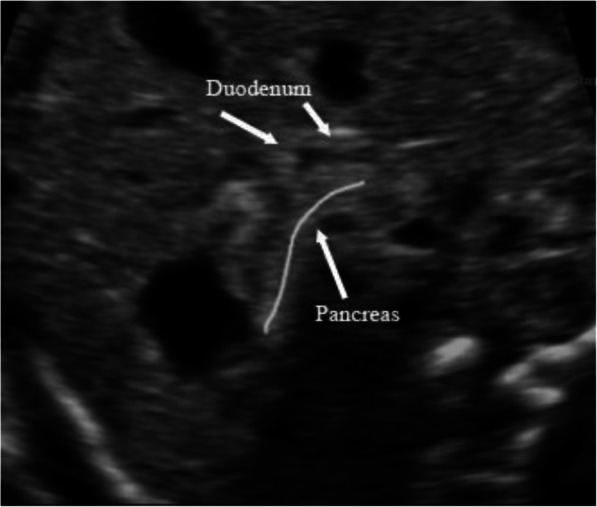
Normal ultrasound images of duodenum and pancreas
